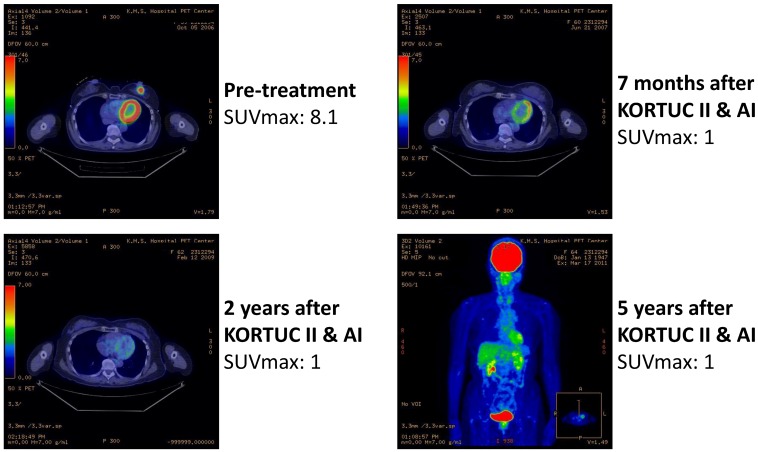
Case 4. 59 years old female patient with left breast cancer (cT2N0M0, ER: +, PgR: +, HER-2: -) treated with radiosensitization (KORTUC II) and without systemic chemotherapy. Left-upper: PET-CT image at pre-treatment. Tumor diameter is 27 mm and SUV-max value is 8.1; Right-upper: PET-CT image at 7 months following KORTUC II. Tumor is not recognized and SUV-max value on the region is approximately 1; Left-lower: PET-CT image at 2 years following KORTUC II. Tumor is not recognized and SUV-max value on the region is approximately 1; Right-lower: Whole body finding of PET-CT image at 5 years following KORTUC II. There is neither local recurrence nor distant metastasis.
